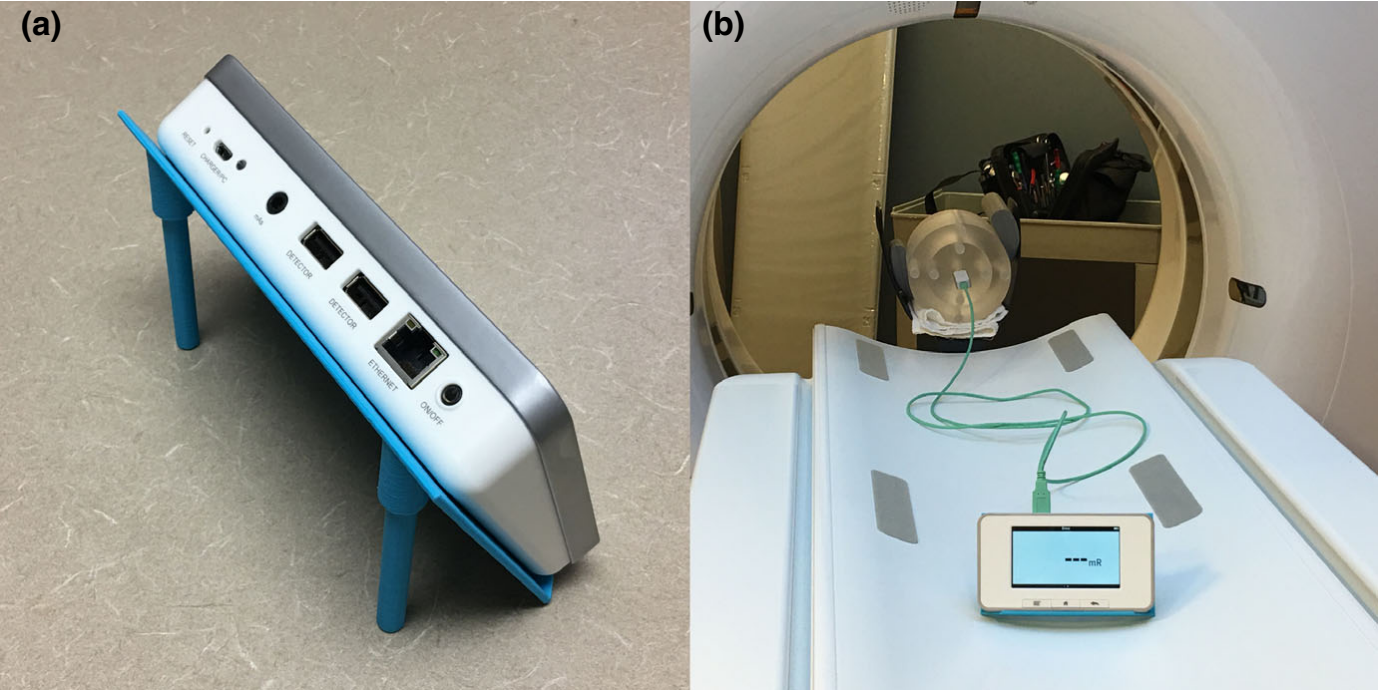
(a) Dosimetry base unit stand, (b) base stand being used in a computed tomography scanner.
